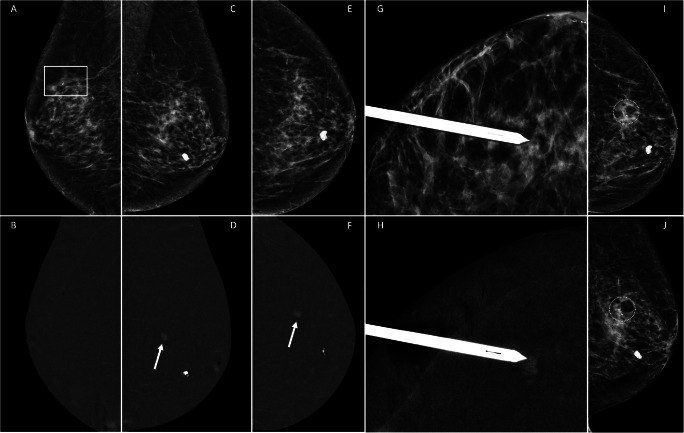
An asymptomatic 77-year-old patient with a history of right breast-conserving surgery was referred for complementary evaluation after detection of a suspicious scar in a routine external centre ultrasound (images not available). Low-energy CEM MLO view (A) of the right breast shows a stable architectural distortion related to the postsurgical scar (rectangle) with no enhancement on the recombined image (B). Left breast recombined images (D and F) show an incidental 7-mm enhancing mass (arrow) not seen on low-energy images (C and E, respectively) or ultrasound. Left breast CEM-guided biopsy was performed (G and H). The correct location of the radiological marker was confirmed, with the usual 2 mammographic craniocaudal and mediolateral views (I and J). Pathology report: intraductal papilloma. The lesion was further assessed with a DBT-guided vacuum-assisted excision (VAE)
